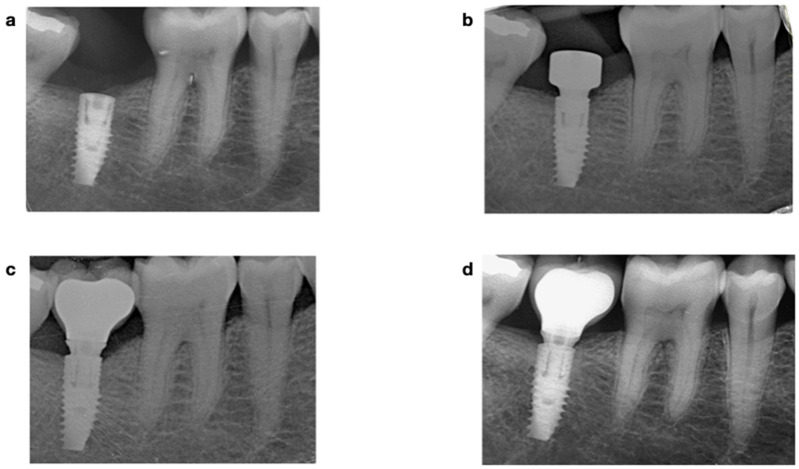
Periapical radiograph-parallel technique for MBL analysis at the 1-year follow-up. (a) 2-stage implant placement; (b) 2-stage surgery 4 months after implant placement; (c) CAD-CAM metallo-ceramic crown cemented to a 0.5 mm anti-rotatory Ti-base abutment at the 6-month follow-up; and (d) at the 1-year follow-up.
